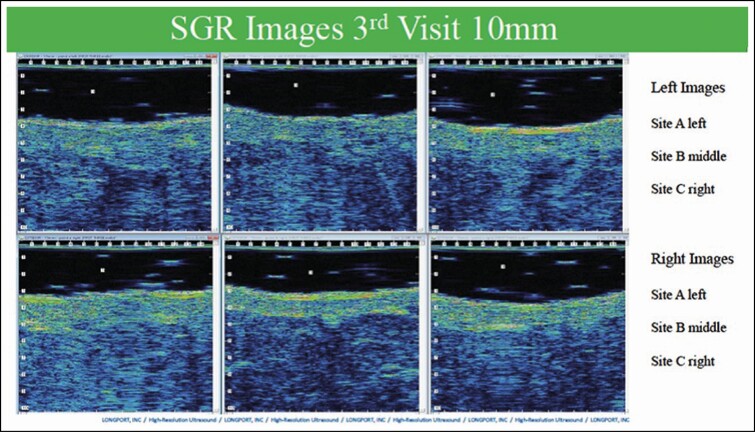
Ultrasound image: bland moisturizer on the top (Left Images) and RSN/TFB on the bottom (Right Images). Percentage change from the dermis to SUBQ L-AVG 127.60 R-AVG 155.1. The dermal image analysis indicates that the right dermis is more echogenic or brighter, suggesting it is denser. Combining the data analysis, the difference is 22%. A typical example of a comparison of fluid disruption into dermal segment representing induration and edema showing less disruption of dermal subcutaneous boundary on RSN/TFB side. TFB, TransFORM Body Treatment with TriHex Technology; RSN, Regenerating Skin Nectar with TriHex Technology.
